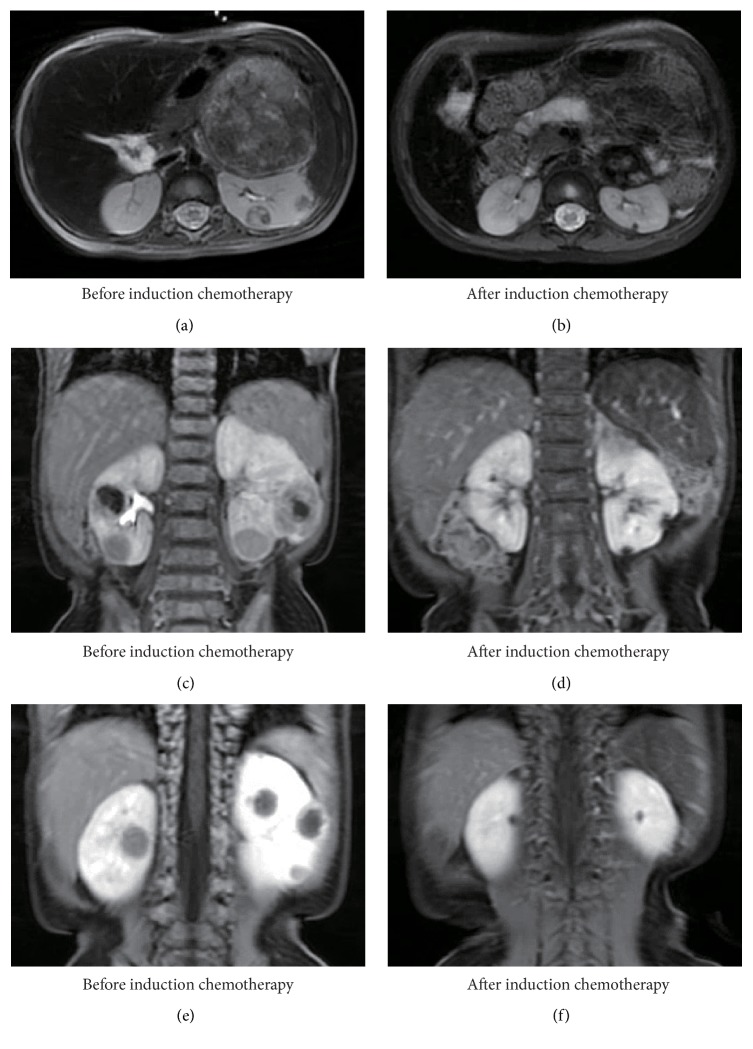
MRI findings before and after induction chemotherapy. Before induction chemotherapy, the T2-weighted axial image (a) and contrast enhanced coronal images (c, e) revealed a left adrenal mass and multiple bilateral renal masses. After induction chemotherapy, the T2-weighted axial image (b) and contrast enhanced coronal images (d, f) showed that the primary tumor and all lesions in bilateral kidneys had decreased in size.
